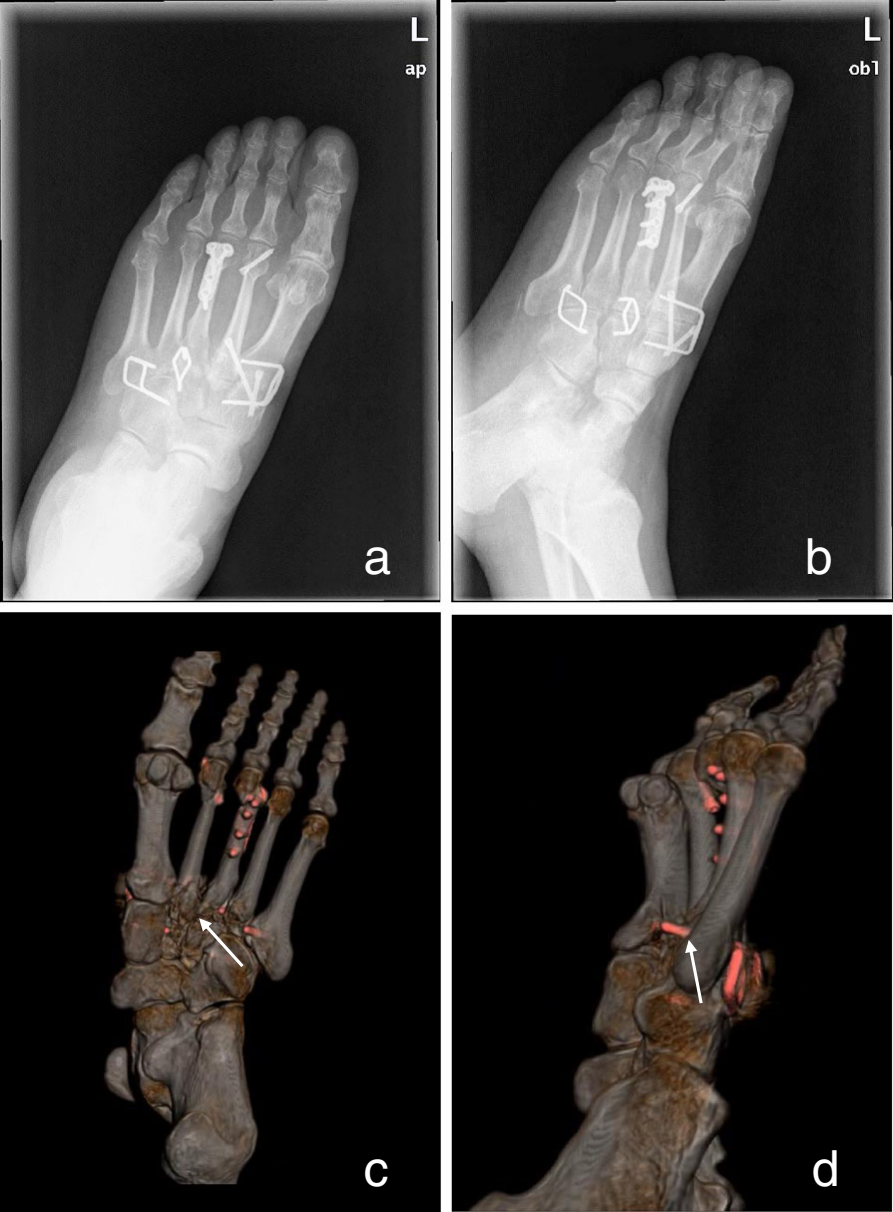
A 50-year-old man with Lisfranc injury of type C2. The anteroposterior and oblique plain radiograph (a, b) showed an anatomical reduction and fine international fixation. But in the VR model of CT images (c, d), a fracture fragment was found poor reduction with a distance of more than 1 mm in image c, while from the lateral view (d), the screw placed into the fifth metatarsal base was found with an exposed part of more than 30% of the screw
